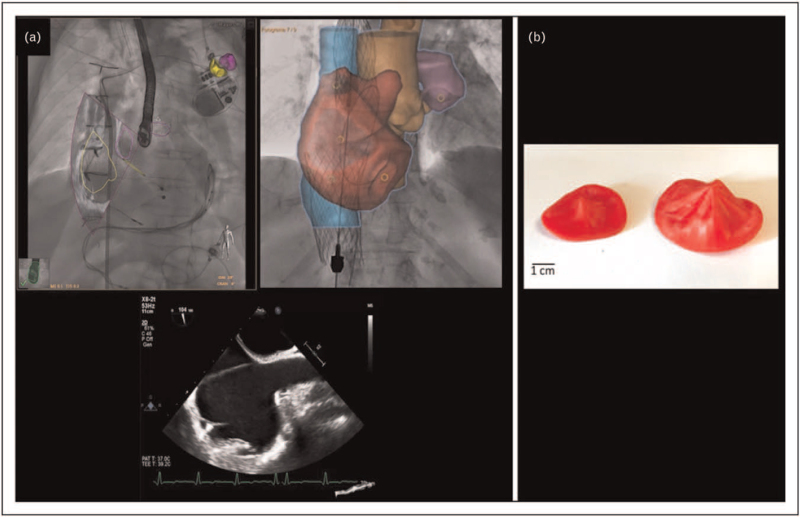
Example of fusion imaging during transcatheter procedure showing transesophageal echocardiographic bicaval view (a, bottom) superimposed on fluoroscopic images (a, top; courtesy of Prof. Jose L. Zamorano, University Hospital Ramon y Cajal, Madrid, Spain). Three-dimensional printing of a normal tricuspid valve (b, left) and a remodeled tricuspid valve (b, right) showing tricuspid annular dilatation and tethering of the leaflets.
